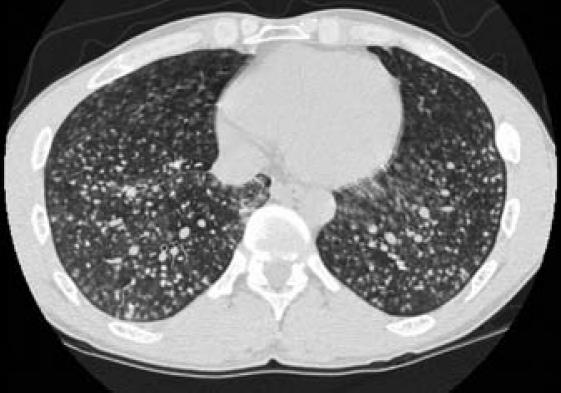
Chest CT scan section of the mid-chest CT showing diffuse
